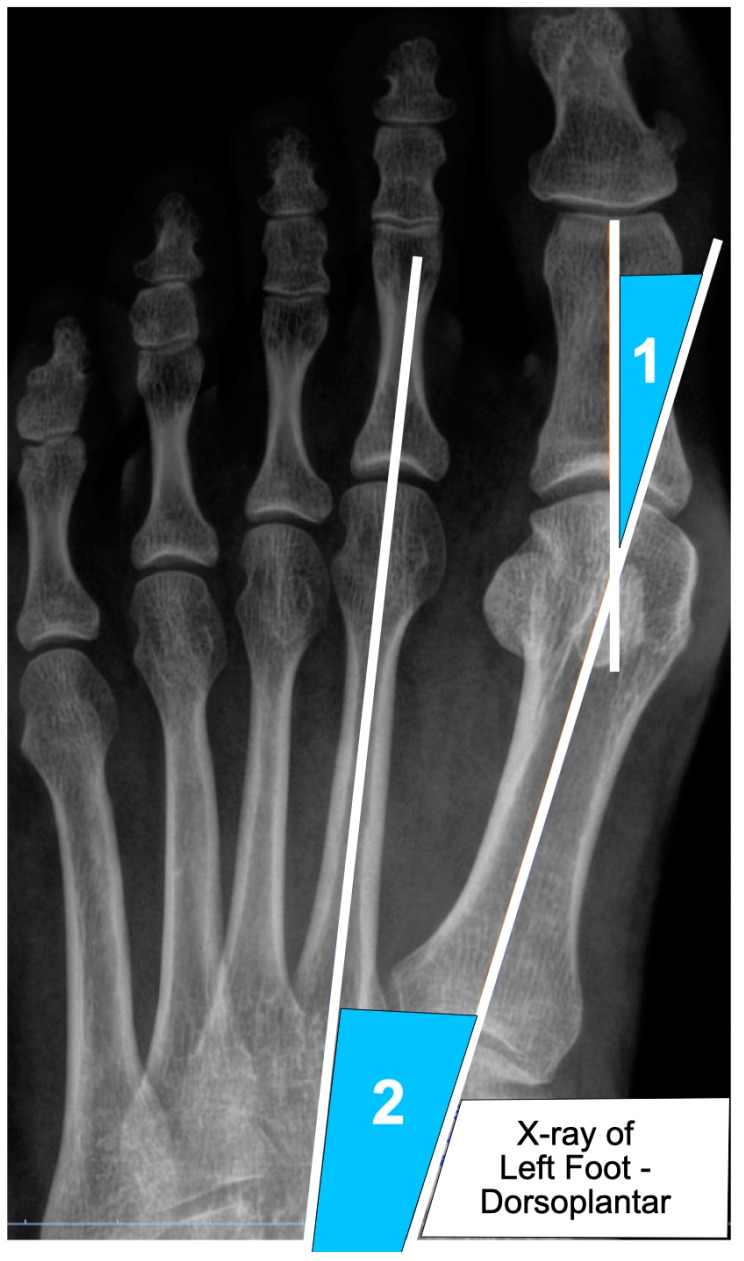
Dorsoplantar X-ray radiograph of the (1) hallux valgus angle (HVA) and (2) intermetatarsal angle (IMA); the HVA is measured as the angle formed between the longitudinal axis of the first metatarsal and the proximal phalanx of the hallux; the IMA is measured as the angle formed between the longitudinal axes of the first and second metatarsal bones.
